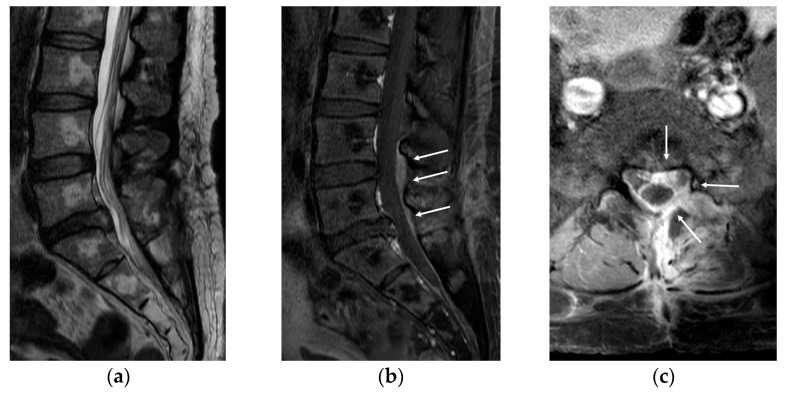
Magnetic resonance imaging (MRI) was conducted to assess the recurrent pain in her back and left lower extremity at 11 days after surgery. (a) MRI revealed no evidence of recurred or remnant disc. (b,c) However, diffuse enhancement was noted at the posterior epidural space from L4 to S1 levels (arrows) and around the peridural space at the L5/S1 level (arrows).
